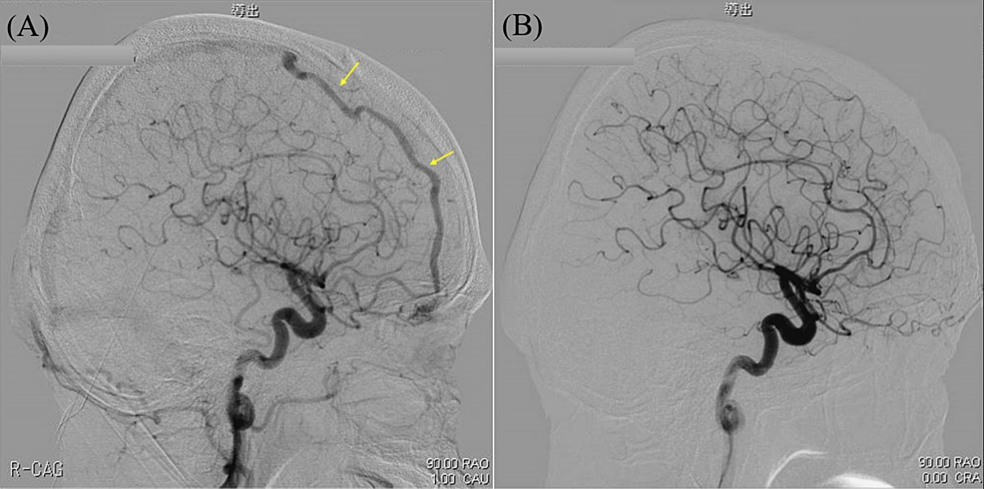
Results of the cerebral angiography (CAG). (A) CAG on admission showing an ethmoidal dural arteriovenous fistula (dAVF) (arrowhead). (B) CAG on six months after operation showing decreased density and thinning of dAVF on radiography but persisted dAVF (arrowhead). (C) CAG on one and a half years after starting immunotherapy and surgical intervention showing the disappearance of the dAVF.
